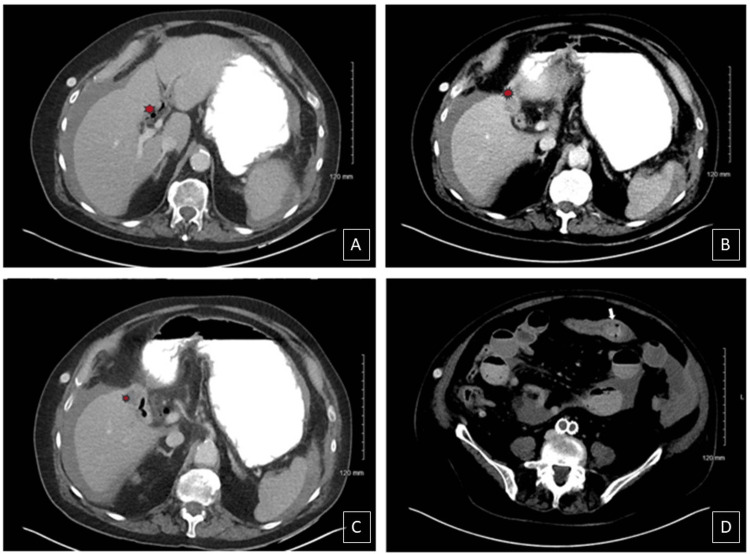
Transverse sections of CT abdomen with contrast on day three of hospitalization A-C: Gallbladder without stone and intraluminal air present (red dot); D: Stone present within the jejunum, distal to the initial position observed in Figure 1 (white arrow)
